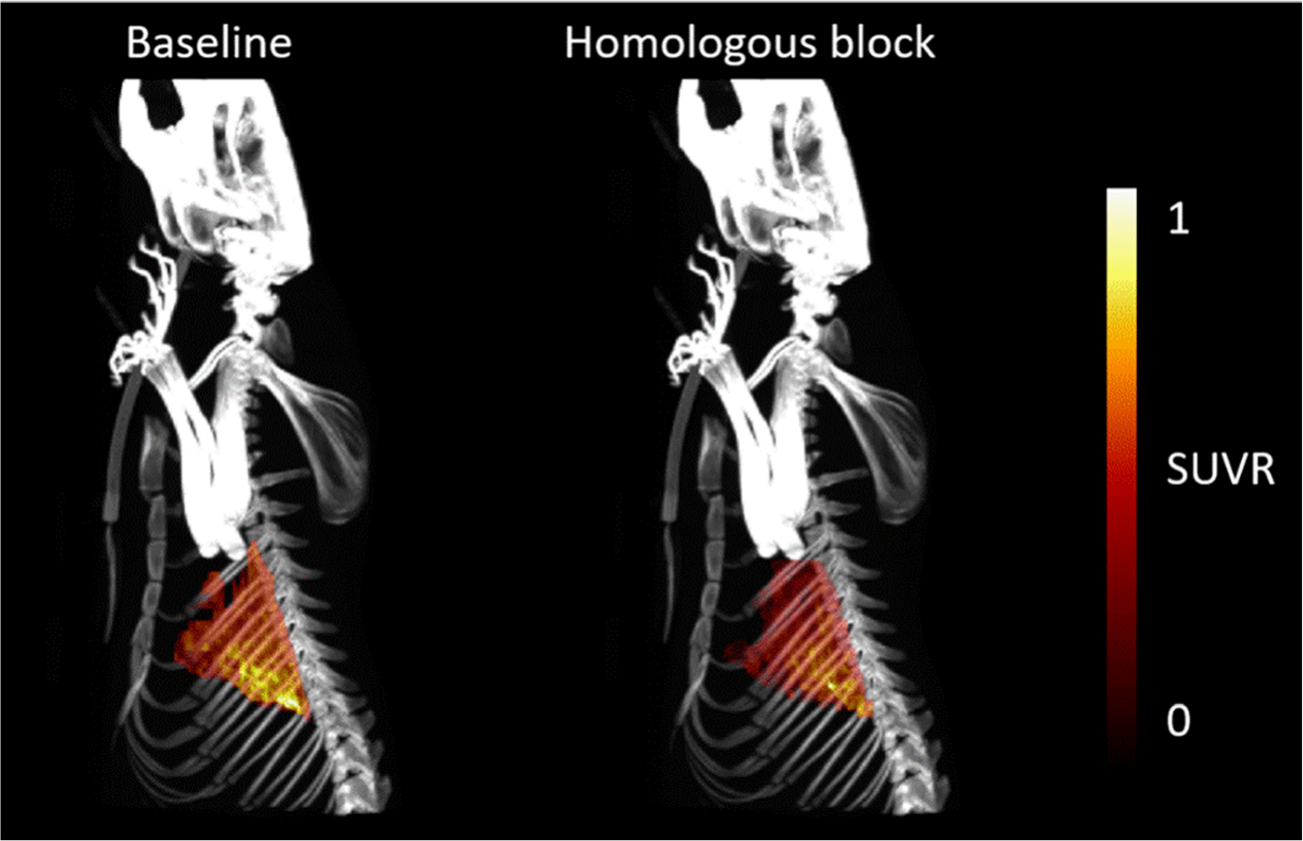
MIP images of co-registered CT and lung-to-heart SUVR PET (30 to 60 min) limited to the lung uptake for subject #2. Left: baseline scan. Right: post-dose scan after administration of FB-A20FMDV2 (2 mg/kg)
